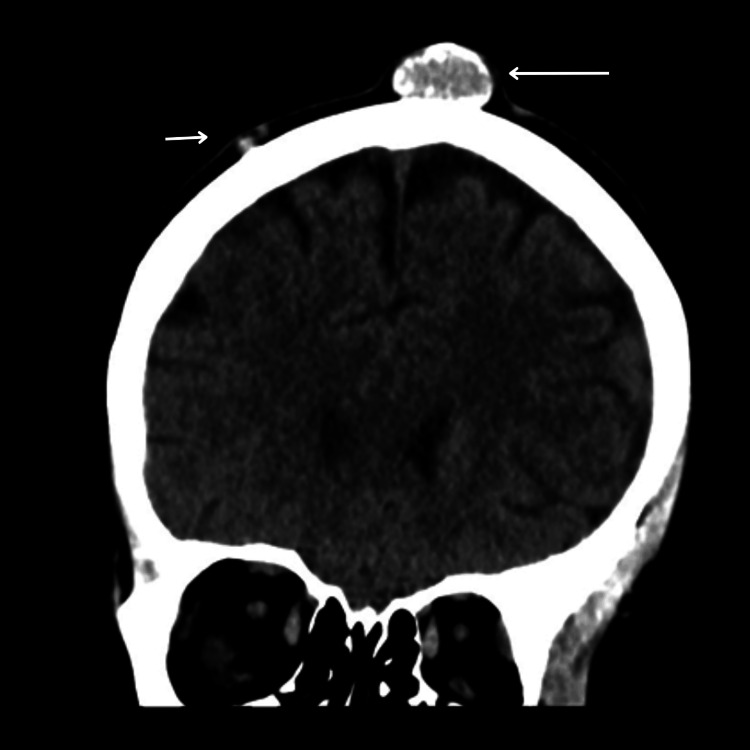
Coronal view of the noncontrasted CT scan of the head A large midline solid cyst with partial calcification is noted. Two small masses in the right parietal area are noted (arrows) CT: computed tomography
